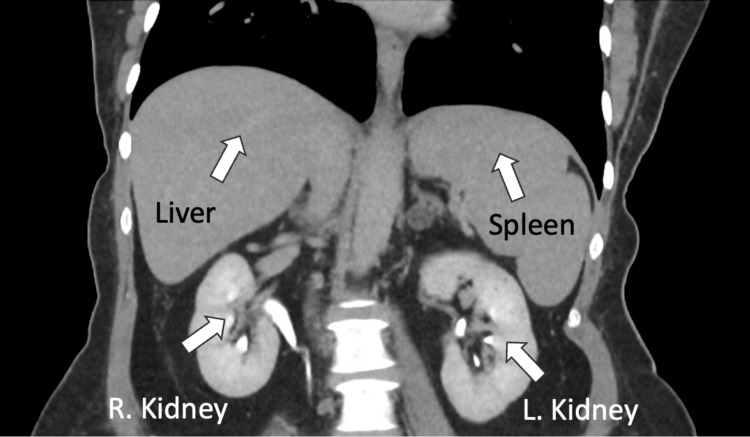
Coronal CT abdomen showing mildly enlarged spleen
